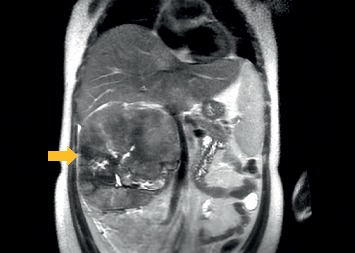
MRI of the abdomen showing a large, right abdominal mass from the unclear origin (arrow).
